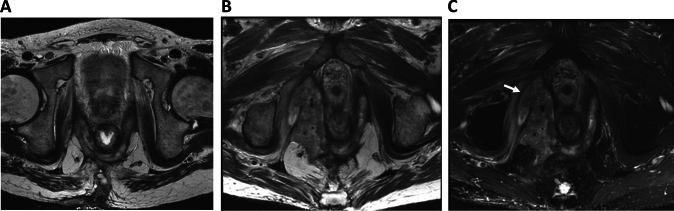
MRI of malignancy complicating perianal fistulizing disease in a patient with Crohn’s disease. A Initial T2W MRI; B, C follow-up T2W MRI (with and without fat suppression). A 42 year old male patient had a history of chronic persistent fistula (A) and refrained from treatment for 5 years. On follow-up MRI a mass lesion is visible, arising within the fistula tract and ending into the supralevator space with evident invasion in the surrounding tissue (arrow)
